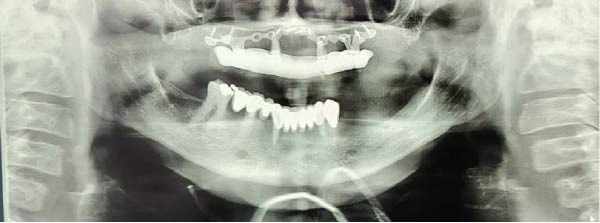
Control orthopantomography.
